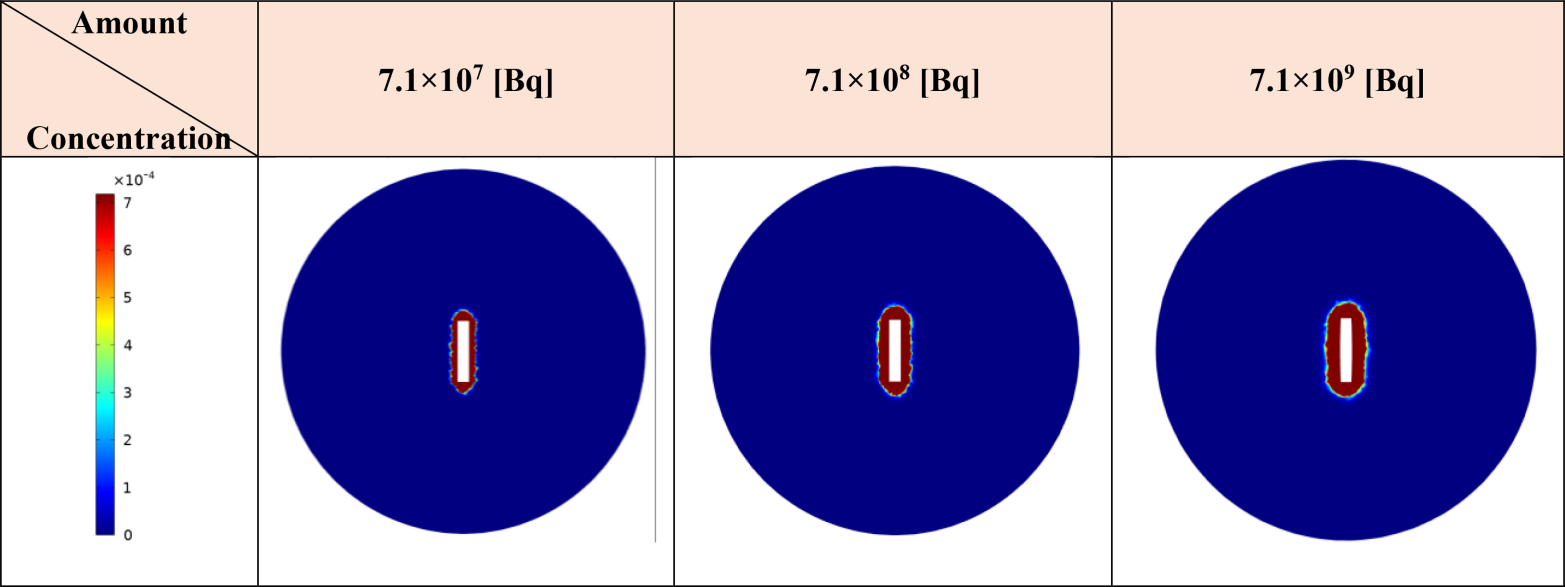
Modifications in the total concentration of released the radiopharmaceutical from implant [MBq/ml] are observed as ^177^Lu-PSMA quantity within the implant increases. Elevating the radiopharmaceutical amount results in a greater accumulation of the drug in the vicinity of the implant.
